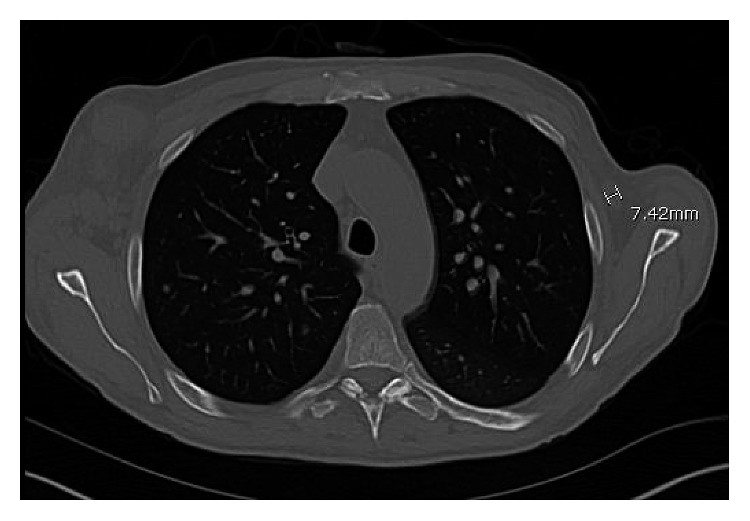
CT chest showing a 5.6 cm × 4.9 cm lymph node mass in the right axilla.
